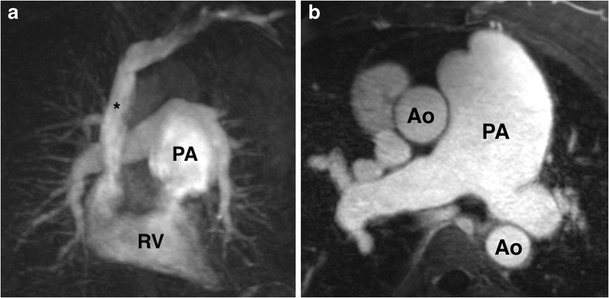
A pulmonary artery MRA in a 55-year-old woman with pulmonary hypertension. Single coronal image from time-resolved pulmonary artery MRA (a) and axial T1-weighted fat-saturated post-gadolinium angiogram image (b) demonstrate fusiform aneurysmal dilatation of the main pulmonary artery (*PA*)
